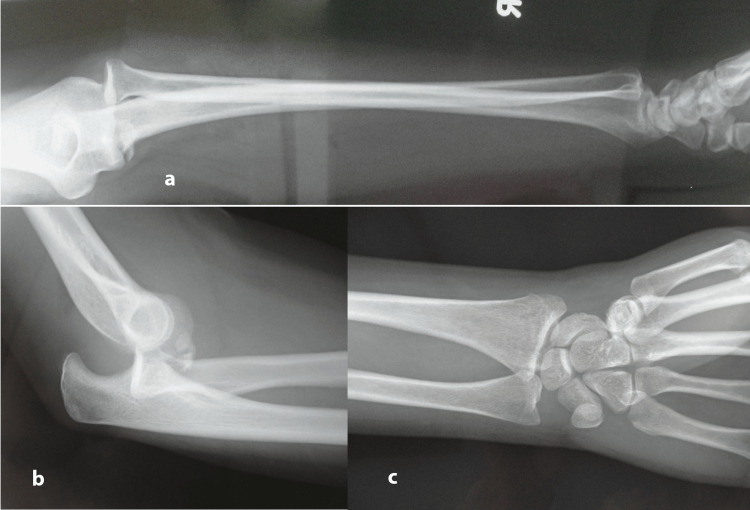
Anteroposterior, (a) lateral elbow, (b) anteroposterior wrist, (c) X-rays at time of injury. Posterior elbow dislocation along with DRUJ dislocation are evident. DRUJ: distal radio-ulnar joint
